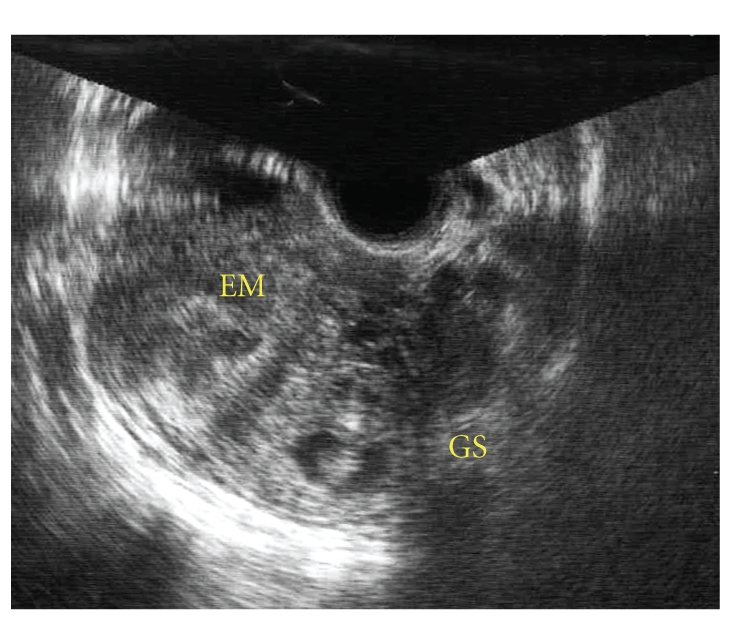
Preoperative ultrasonographic image. EM: endometrium, GS: gestational sac. GS was distinct from EM at the left side of the uterus.
